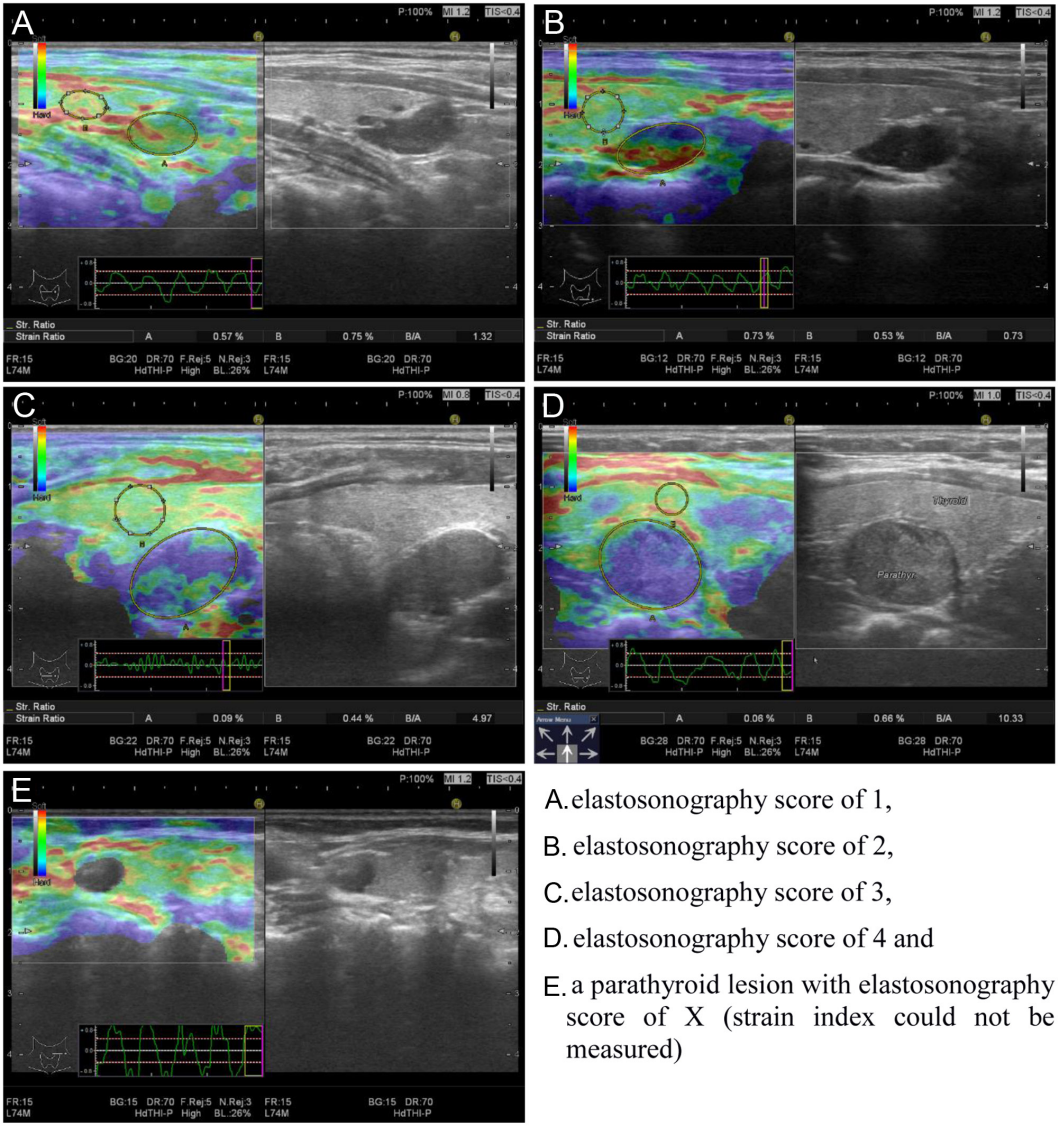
Ultrasound elastography score and strain index measurements in parathyroid lesions.
